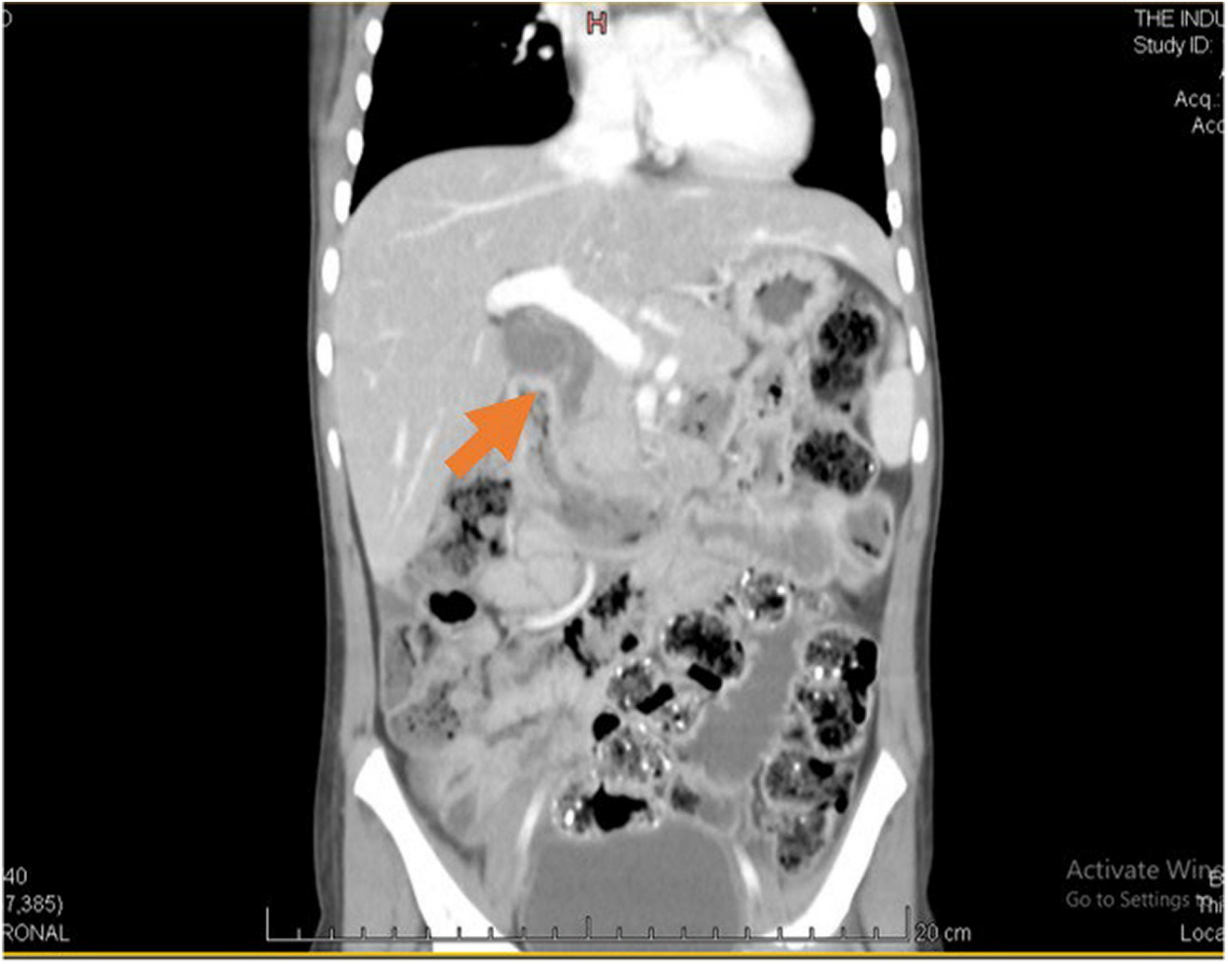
CT abdomen of the patient (coronal view).
